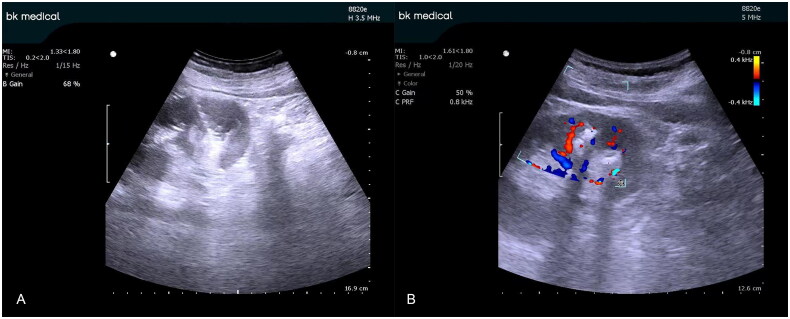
B-ultrasound imaging (A) and color Doppler ultrasound imaging (B) of the same nonhydronephrosis while performing percutaneous renal puncture. Red indicates the artery; blue indicates veins.
